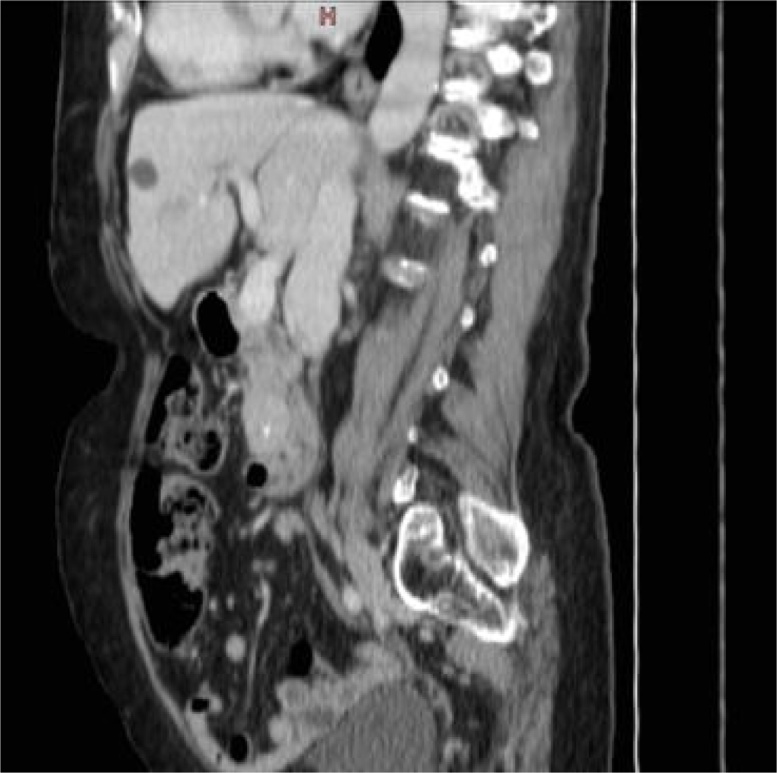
Abdominal CT, sagittal section: tumor mass of the pancreatic uncinate process.
